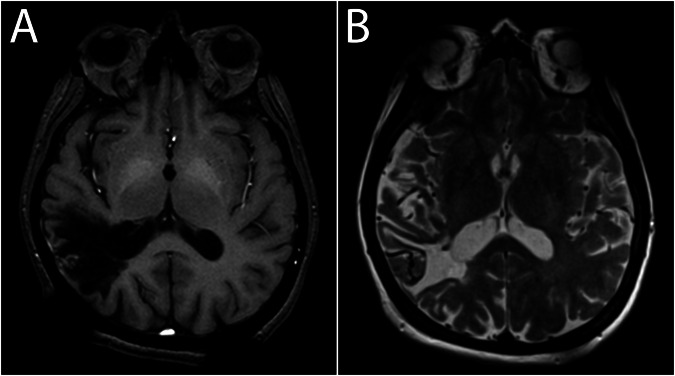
Hyperintensity in the GP in a patient suffering from liver cirrhosis. A non-contrast T1-weighted image displays symmetric hyperintensities in the globi pallidi. B T2-weighted image shows expected hypointensity in the globi pallidi. Additional T2 shortening due to the present T1 shortening cannot be appreciated by the naked eye. The peritrigonal lesion on the right side is a posthemorrhagic pseudocyst due to arteriovenous malformation
